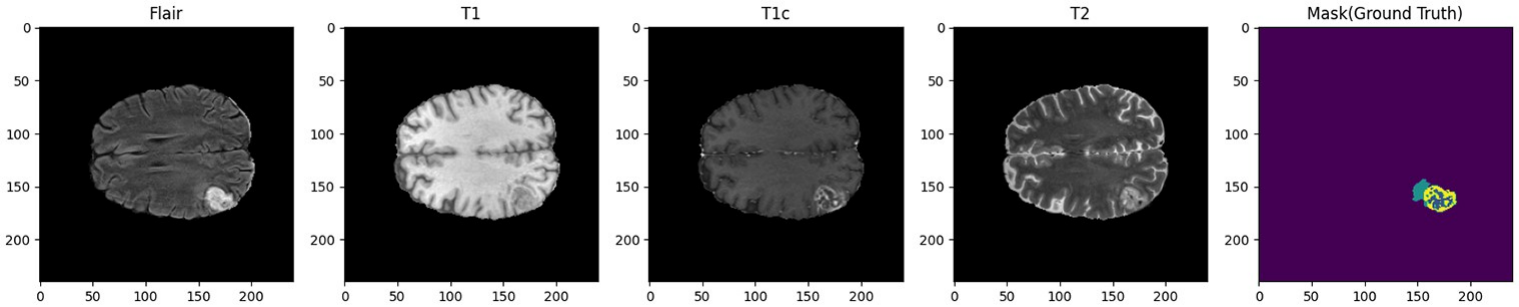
MRI sequences as 2D sections.
